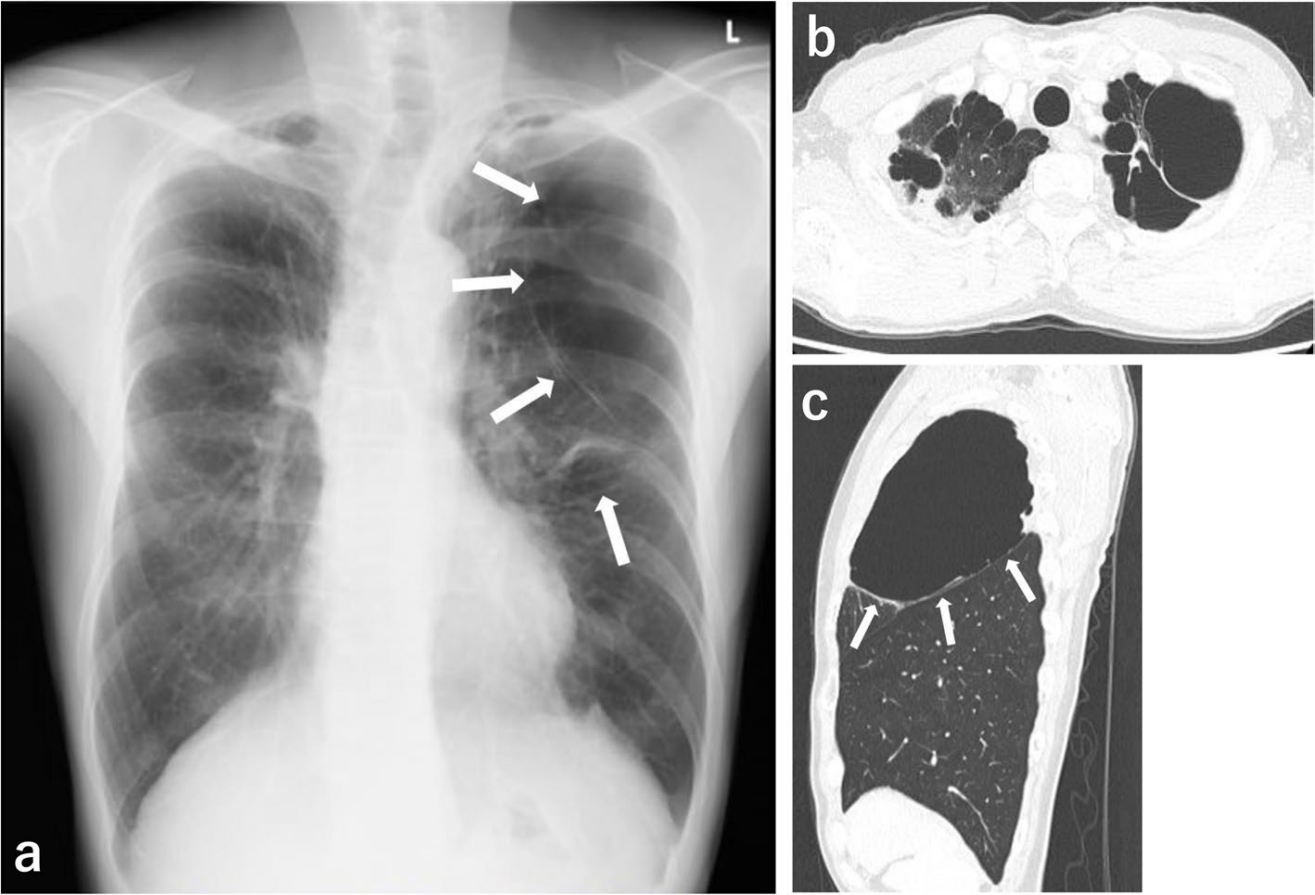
Pre-operative chest radiography (a) showing bulla on the left side (*arrows*). Horizontal slice (b) and sagittal slice (c) of computed tomography showing bilateral diffuse emphysematous changes, multiple bullae in the right upper lobe, and a giant bulla, in the left upper lobe (*arrows*)
